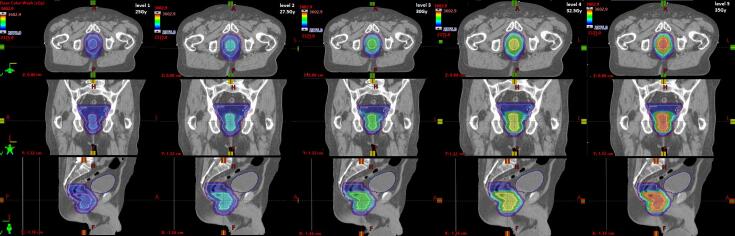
Example of study patient planning CT showing dose colour wash at baseline 25 Gy/5# (level 1) and dose levels 2–5 (27.5 Gy, 30 Gy, 32.5 Gy and 35 Gy) with increasing dose to PTV_High.
